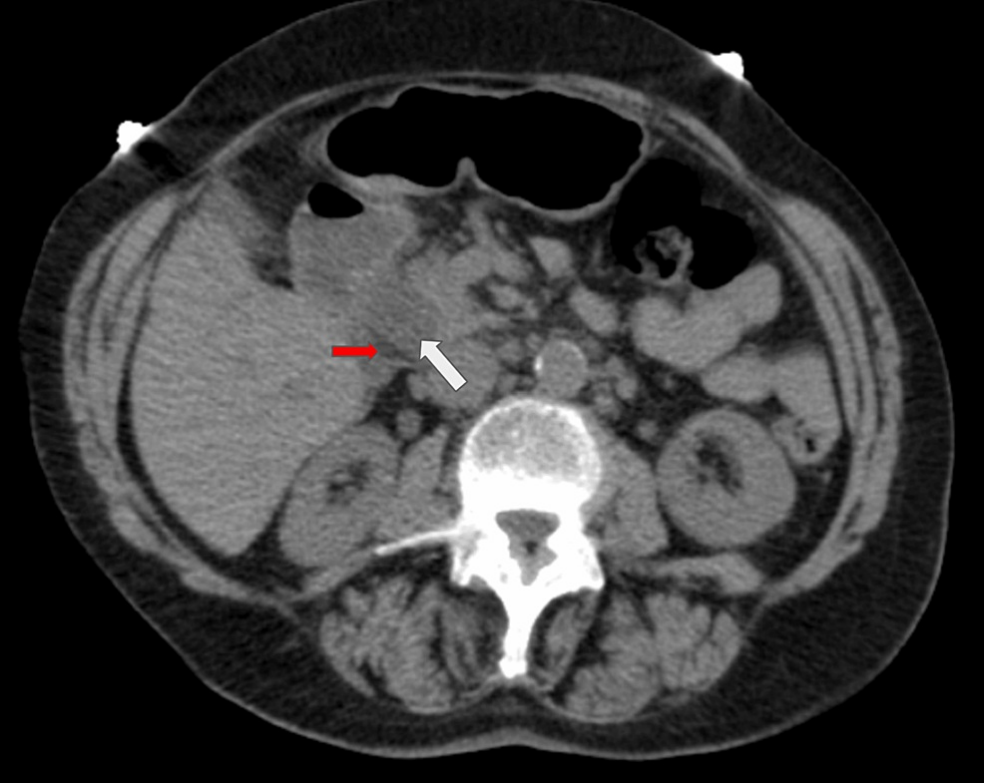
Axial CT of the abdomen without contrast shows intrapancreatic CBD dilation (28mm). The white arrow depicts a non-radiopaque calculus creating an incomplete obstruction of the bile duct. The red arrow depicts bile in the non-obstructed portion of the CBD. CBD: common bile duct
